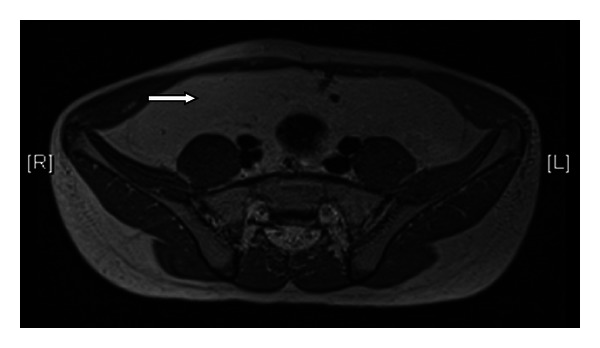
MRI pelvis: lipomatous tissue in the pelvis.
